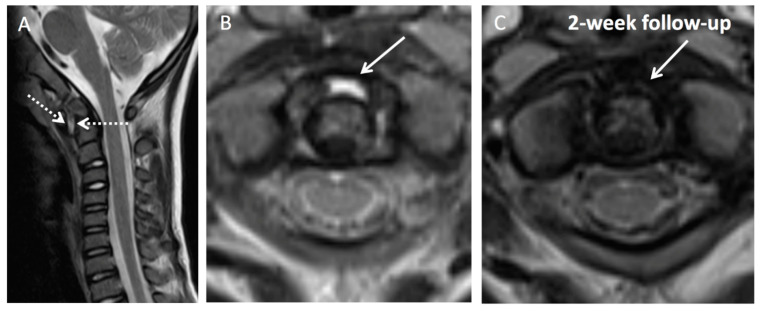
MRI shows edematous swelling of the posterior naso-, oro- and hypopharyngeal soft tissue with extension to the cruciate ligament (A); 2D T2w TSE. Joint effusion in the anterior synovial compartment of the dens seen as high T2 signal (B); arrow; 2D T2w TSE. No abscess formation present. Forward movement of C1 with increased anterior atlantodental interval (A); dotted arrows. No spinal cord compression; 2-week follow-up with appropriate empiric antibiotic regimen shows full regression of joint effusion (C); 2D T2w TSE. T2w = T2-weighted; TSE = turbo spin echo; T1w = T1-weighted; CA = contrast agent.
